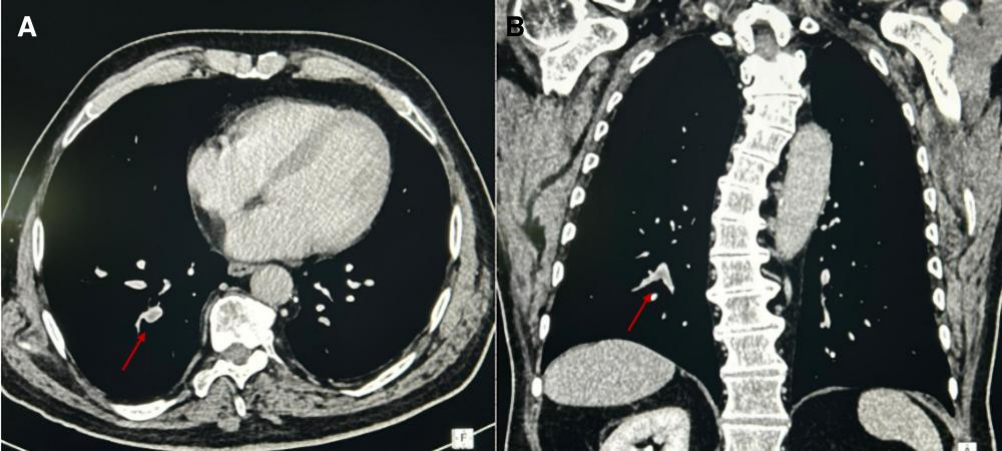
Computed tomography pulmonary angiography showing multiple filling defects in the segmental branches of the pulmonary arteries, consistent with pulmonary embolism (*A* and *B*, arrows; coronal and sagittal planes, respectively). CTPA, computed tomography pulmonary angiography.
